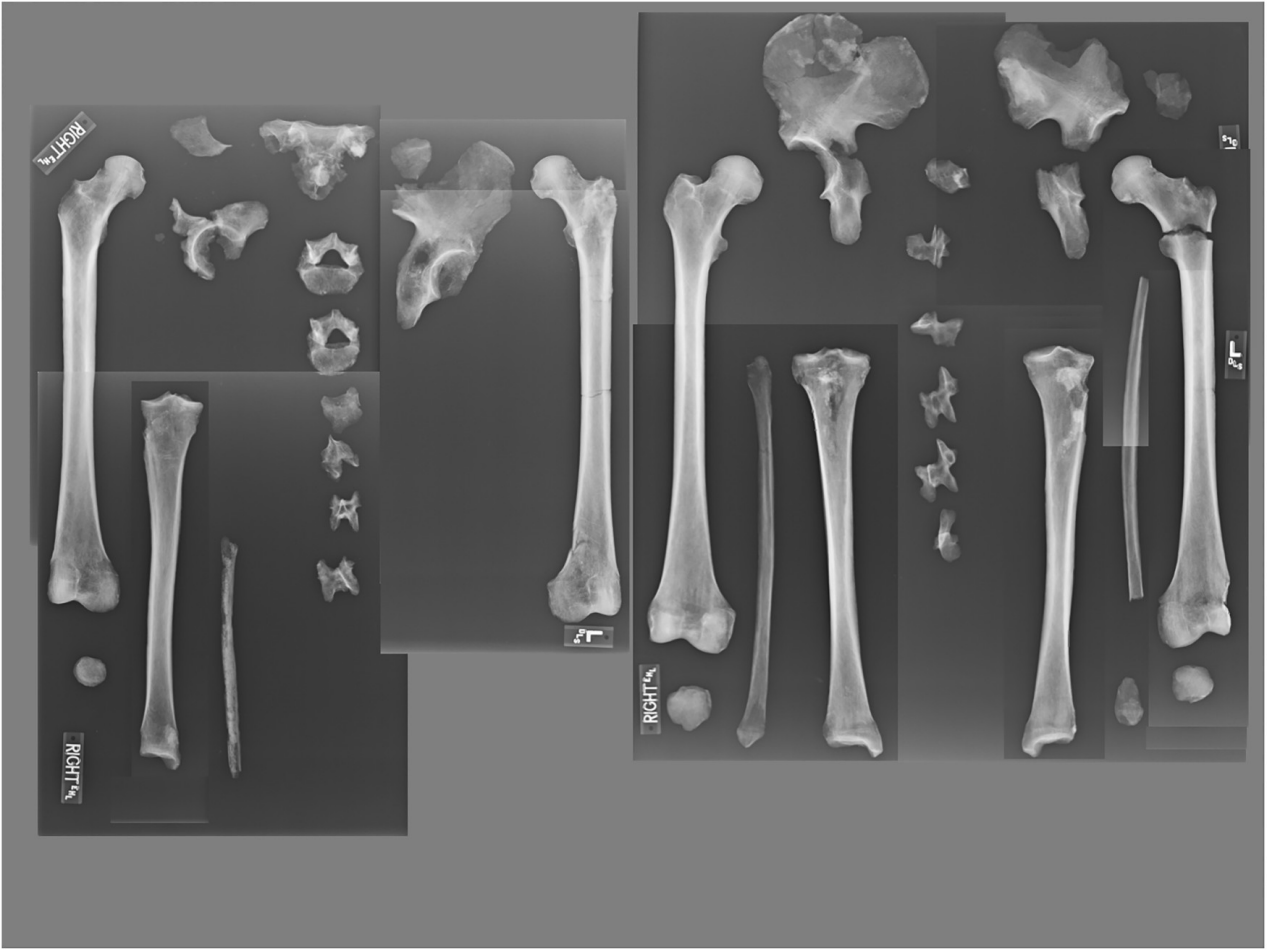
Comparison of YNH4 B (left) and B2 (right) lower limb radiographs. Note relatively higher translucence and lower bone density in B relative to B3. See text for details.
